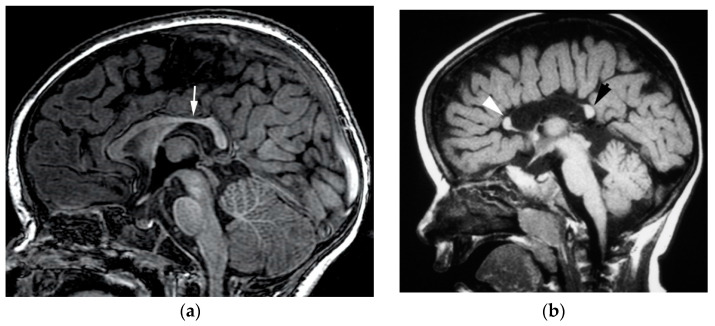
(a): Normal midline sagittal T1-weighted image of fully developed corpus callosum. Note the slight thinning of the posterior body (white arrow), which is normal. (b): Midline sagittal T1-weighted image of corpus callosum agenesis. The two midline commissures seen anteriorly and posteriorly are the anterior commissure (white arrowhead) and hippocampal commissure (black arrow) respectively. These would not be present in tricommissural agenesis.
